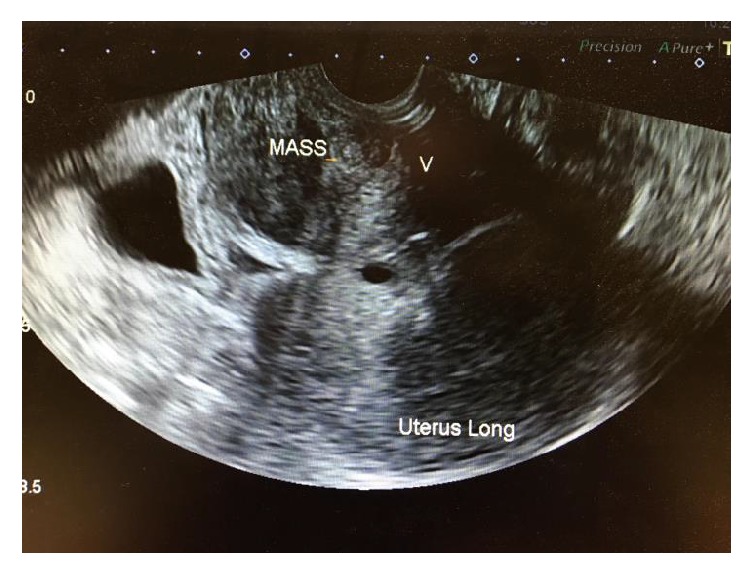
Ultrasound scan showing relation of mass to uterus and vagina.
